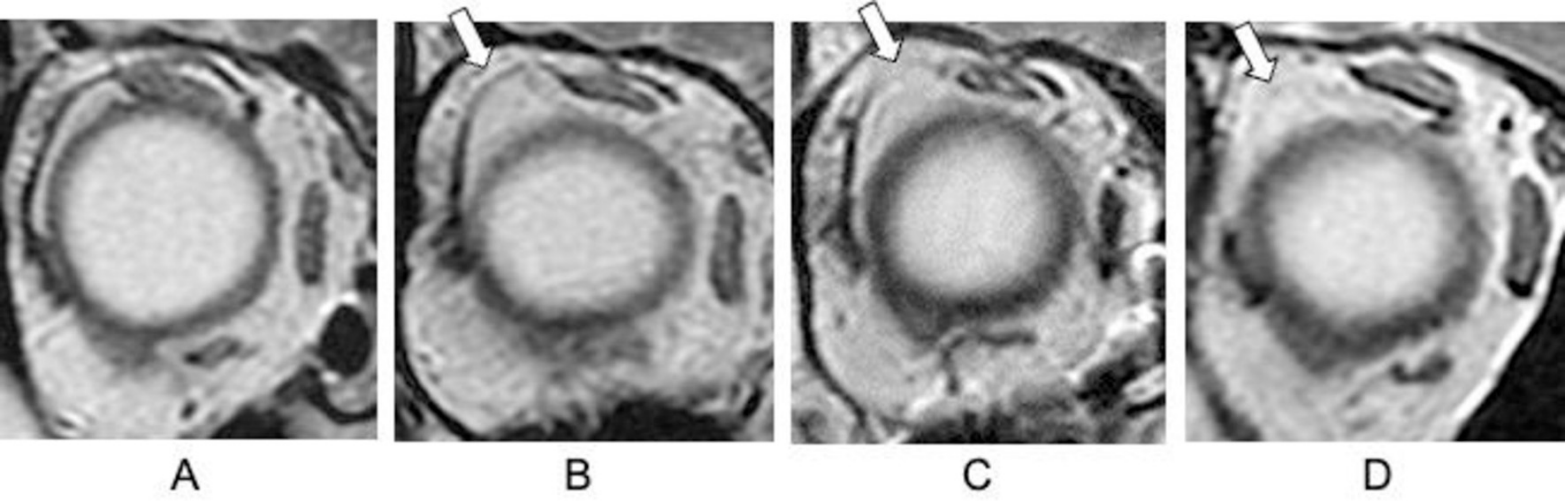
Details of the lateral rectus–superior rectus band evaluation by orbital coronal magnetic resonance imaging. (A) Control. (B) Elongation. (C) Rupture. (D) Disappearance.
